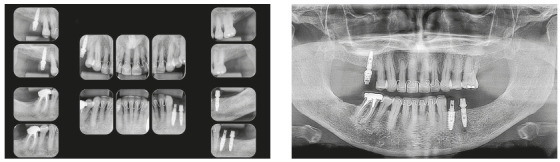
Final radiographs of Case 2.
